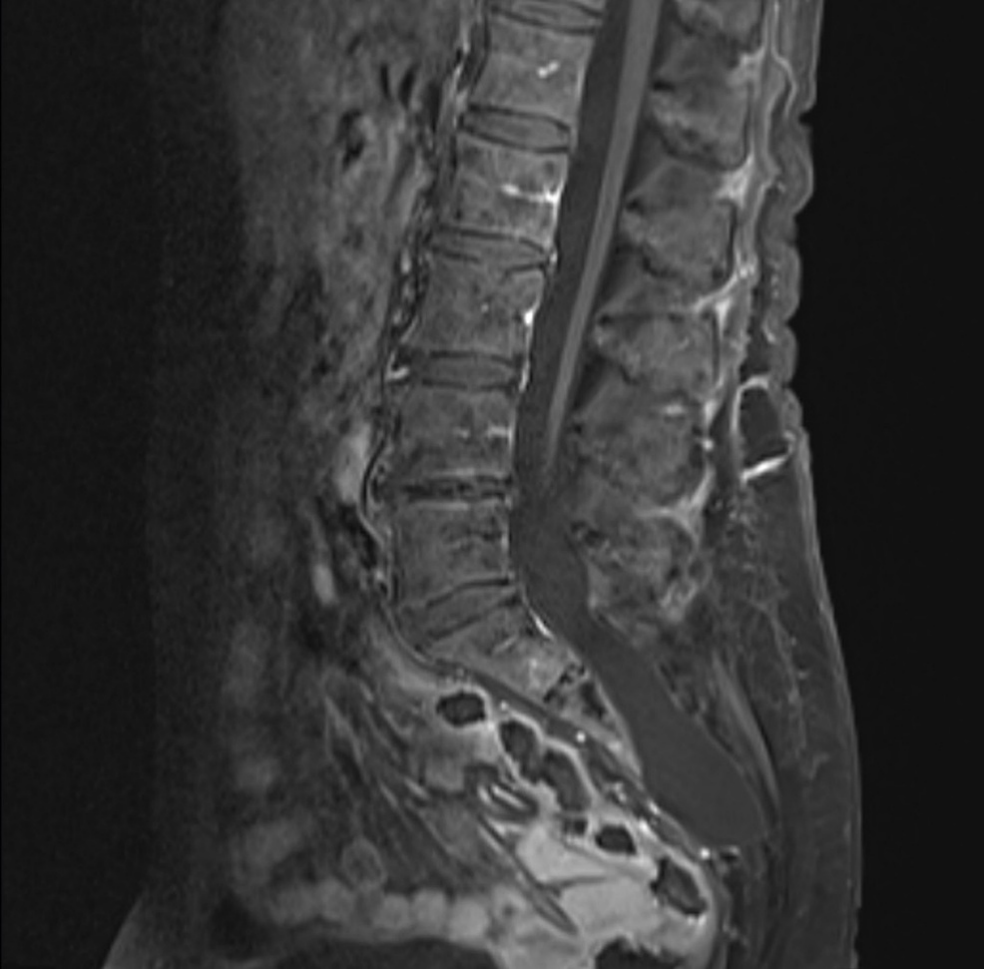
Postoperative six-month follow-up. Sagittal T1-weighted MRI with contrast revealing complete resolution of the intraspinal hemorrhage, with expansion of the sacral spinal canal and cystic dilation.
